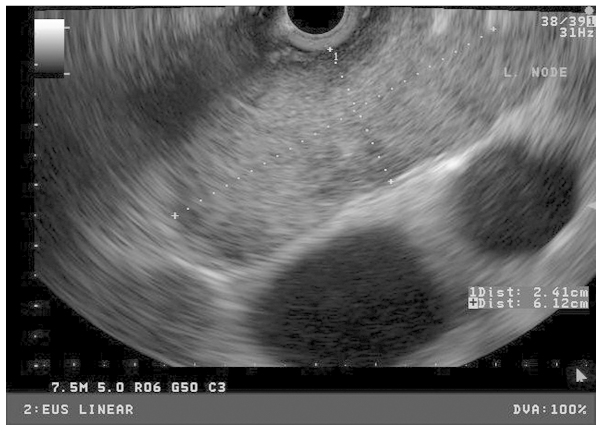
Endoscopic ultrasound of the largest mediastinal lymph node, located in the subcarinal region, measuring 2.41×6.12 cm in diameter.
